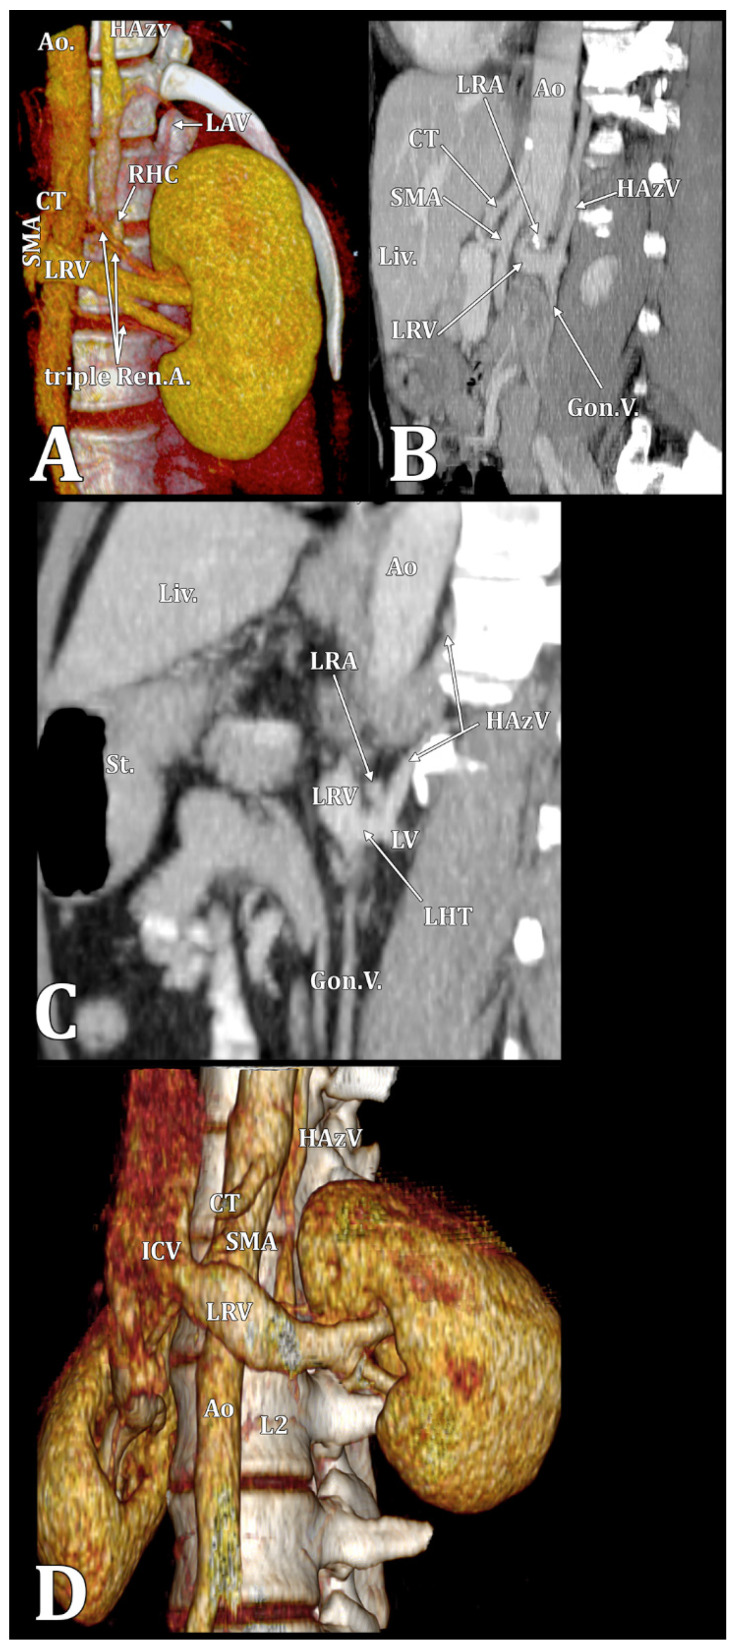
Type 1 RHC cases. (A) A triple left renal artery was found in a type 1 male case. In that case, the RHC had a 9.56 mm caliber, and the LRV was 9.61 mm. Therefore, the LRV and RHC were almost equal in size. The upper and middle left renal arteries crossed the RHC anteriorly. Three-dimensional volume rendering of the left kidney and renal vessels, anterior view. Ao., aorta; CT, celiac trunk; HAzV, hemiazygos vein; ALV, lumbar ascending vein; LRV, left renal vein; Ren.A., renal artery; RHC, reno-hemiazygos connection; and SMA, superior mesenteric artery. (B) In a type 1 female case, the LRV crossed the aorta anteriorly at the level of the L2 vertebra. Immediately after crossing the aorta, the LRV connected an RHC posteriorly and, inferiorly, the left gonadal vein. The RHC ascended posterior to the left RA and continued as an HAzV on the posterior side of the aorta. Sagittal angioCT slice. Left lateral view. Ao, aorta; CT, celiac trunk; Gon.V., left gonadal vein; HAzV, hemiazygos vein; Liv., liver; LRA, left renal artery; LRV, left renal vein; and SMA, superior mesenteric artery. (C) In a type 1 RHC female case, the second left lumbar vein and the RHC were inserted into the LRV as a common lumbar-hemiazygos trunk, immediately inferior to the left renal artery at the L1/L2 intervertebral disc level. Sagittal angioCT slice. Left lateral view. Ao, aorta; Gon.V., left gonadal vein; HAzv, hemiazygos vein; LHT, lumbar-hemiazygos trunk; Liv., liver; LRA, left renal artery; LRV, left renal vein; LV, lumbar vein; and St., stomach. (D) In a type 1 RHC female case, the HAzV had a 6.8 mm caliber. Three-dimensional volume rendering. Left antero-infero-lateral view. Ao, aorta; CT, celiac trunk; HAzV, hemiazygos vein; ICV, inferior cava vein; LRV, left renal vein; and SMA, superior mesenteric artery.
